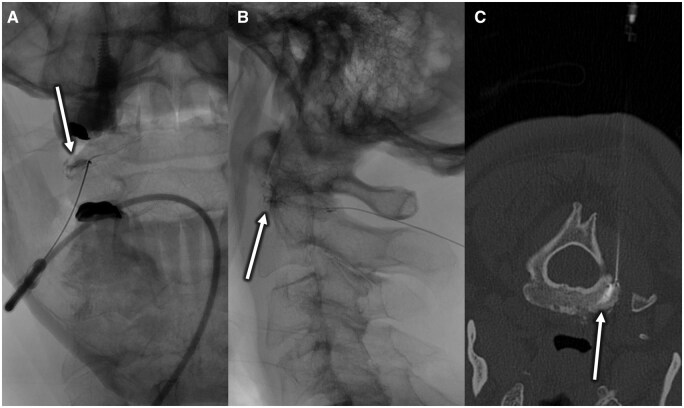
AP (A) and lateral (B) fluoroscopic views demonstrating placement of a needle within the lateral third of the left lateral atlantoaxial joint (patient lying prone) with contrast noted filling the joint space (arrows). Axial CT-guided (C) intraarticular placement of a 25-gauge needle into the lateral atlantoaxial joint with contrast filling the joint space (arrow).
